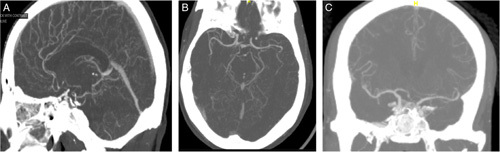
(A–C) Computed tomography angiogram of the brain showed multifocal stenosis in anterior cerebral artery, posterior cerebral artery, and middle cerebral artery territories.
